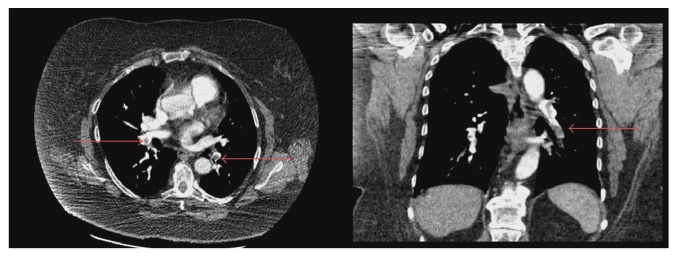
CT angiography showing bilateral pulmonary emboli (red arrows).
